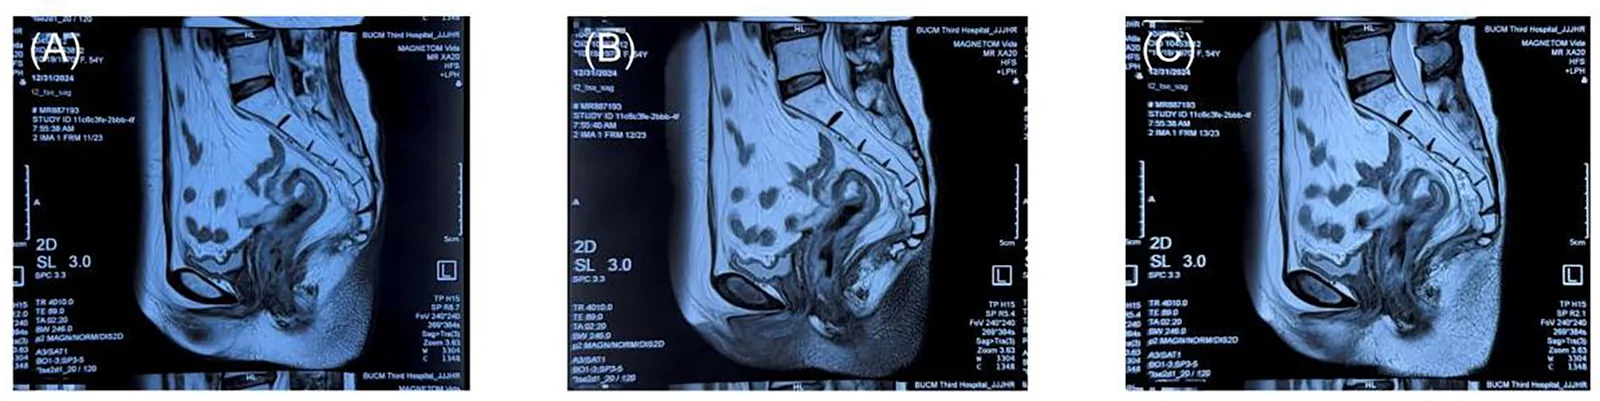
The patient underwent evaluation after completing two cycles of treatment with bevacizumab combined with irinotecan and capecitabine. (A–C) High-resolution pelvic MRI demonstrated that, compared with previous measurements, the degree of rectal wall thickening was significantly reduced, with the maximum thickness measuring 10 mm.
